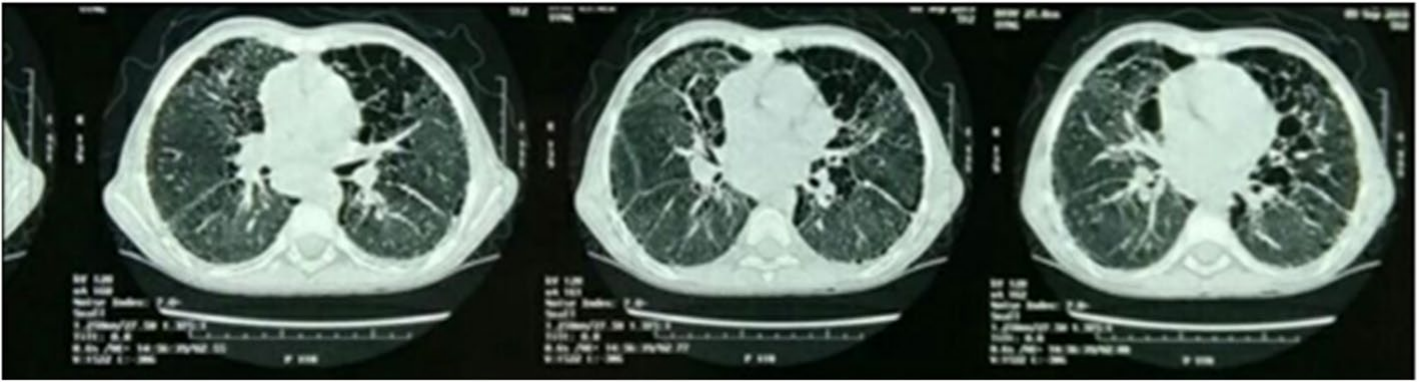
Ground glass appearance, peribronchial thickening, and cyst formation bilateral (HRCT patient number 5)
